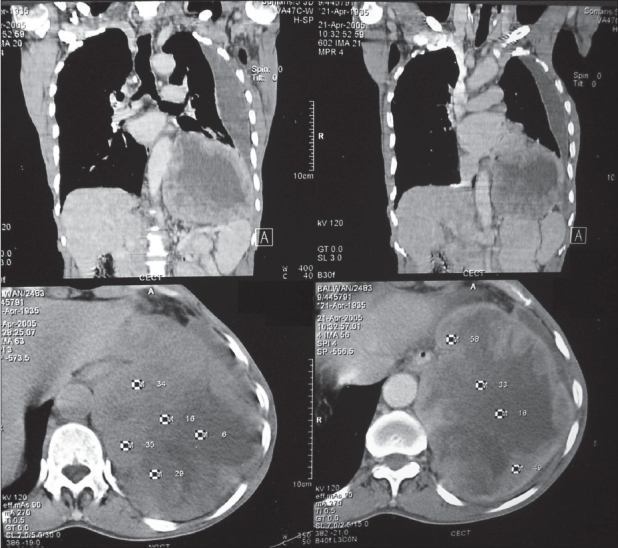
Non contrast and contrast enhanced CT scan images in axial orientation and coronal reformatted images
